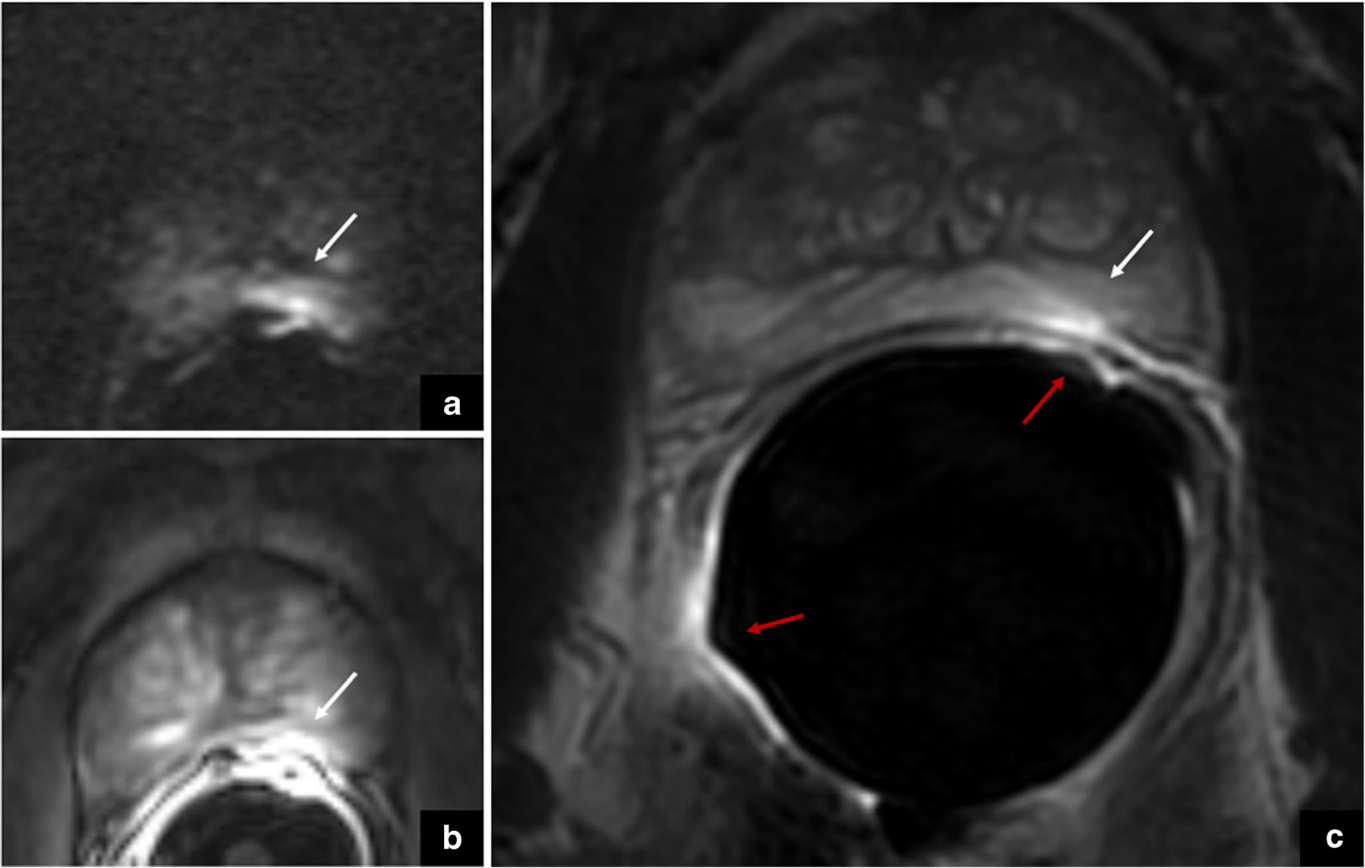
The white arrows show an area mimicking restricted diffusion on DWI (a) and focal enhancement on DCE imaging (b) in the left peripheral zone, very close to the prostatic capsule and adjacent to the endorectal coil surface. This finding could be erroneously interpreted as a lesion, but the axial T2-weighted image (c) does not show this artefact, and therefore prostate cancer can be ruled out. Red arrows show the coil surface
